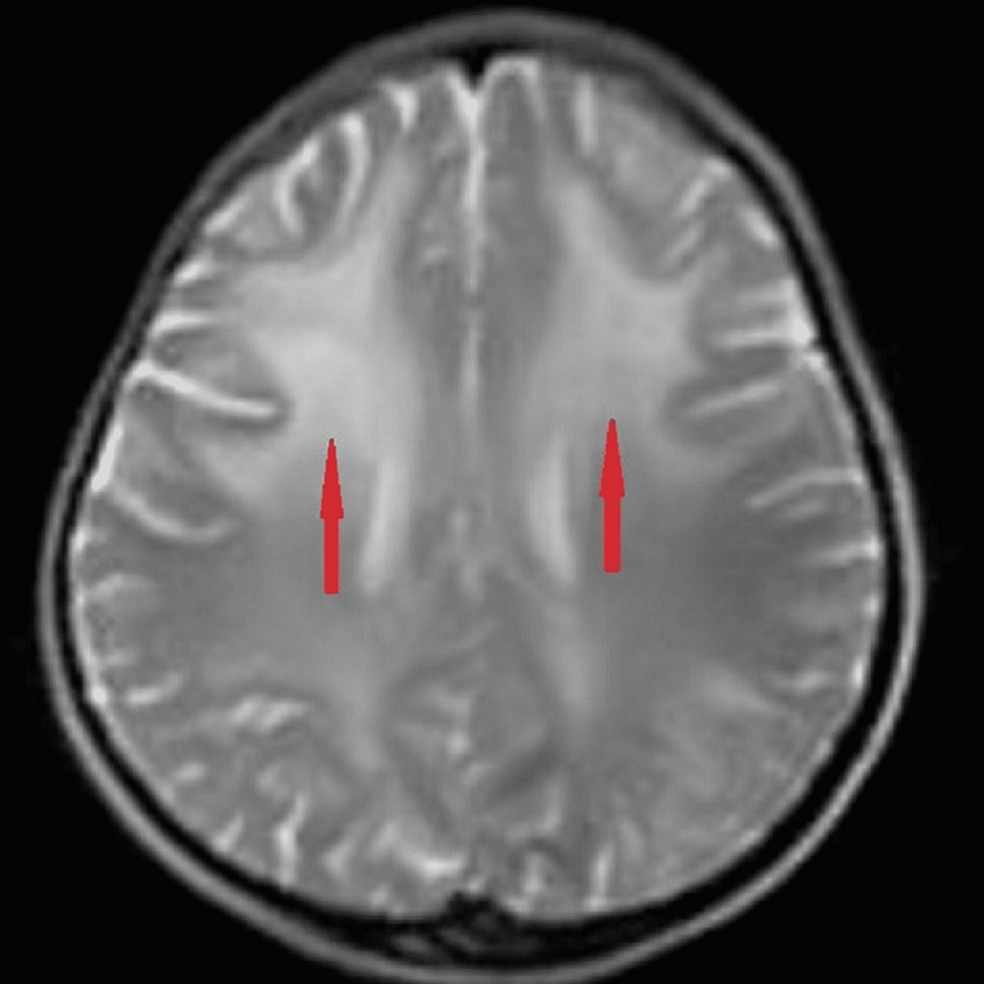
T2W image shows diffuse symmetrical, bilateral hyperintensities with a preponderance of frontal lobes (red arrows). T2W image: T2-weighted image
